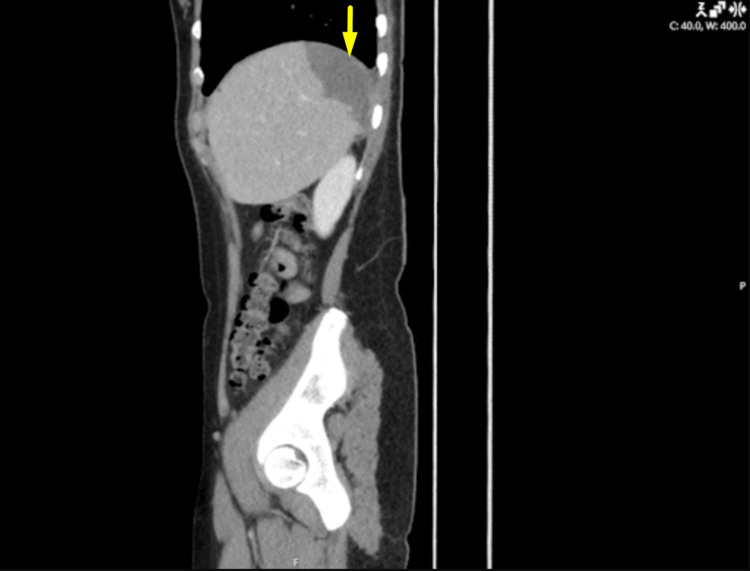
Sagittal CT image of the abdomen Subcapsular wedge-shaped hypoenhancing area at the splenic dome, consistent with an infarct (indicated by arrow).
